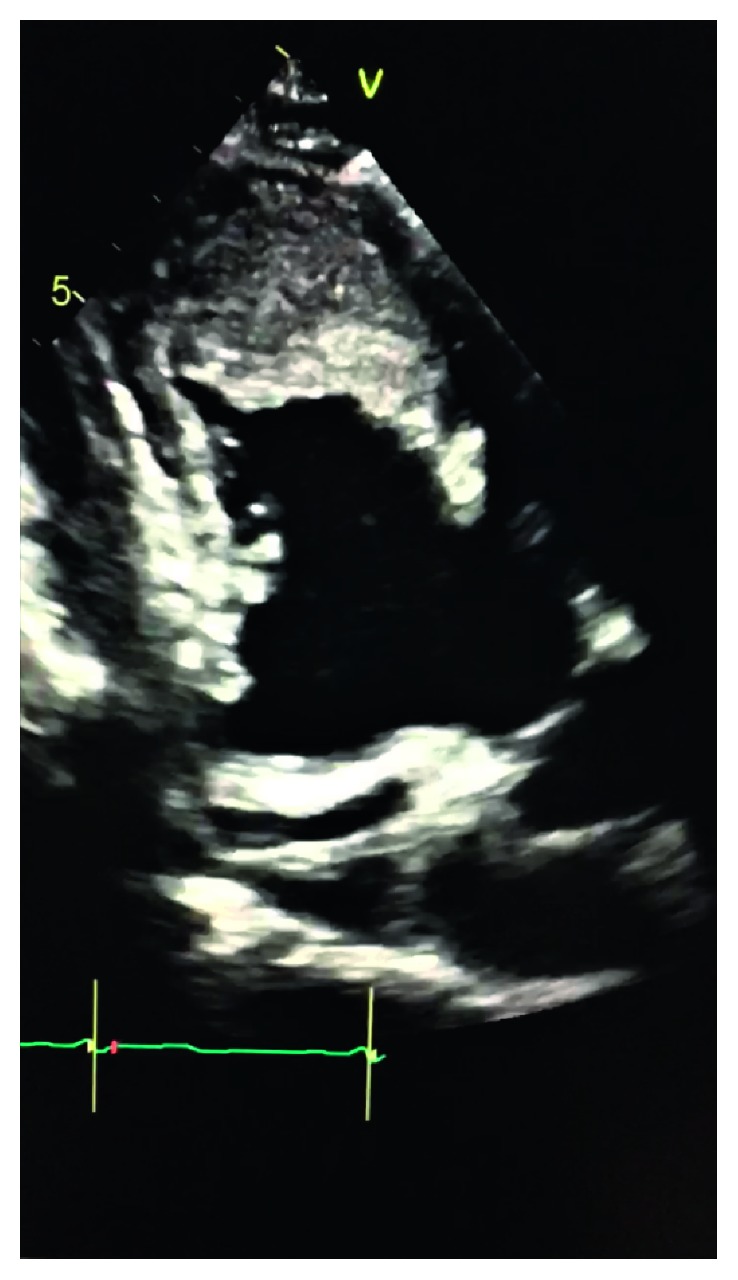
Transthoracic echocardiogram showing the left ventricle filled with thrombus.
